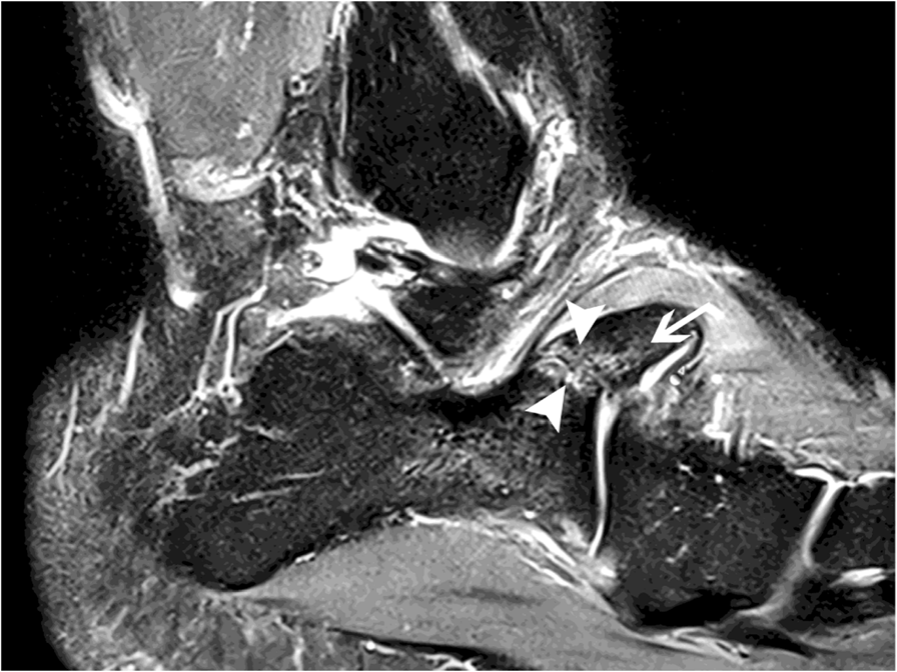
Sagittal T2-Fatsat MR image of the right foot. The arrow show the calcaneus secondarius with T2 hyperintensity corresponding to synchondrosis edema (arrowheads)
